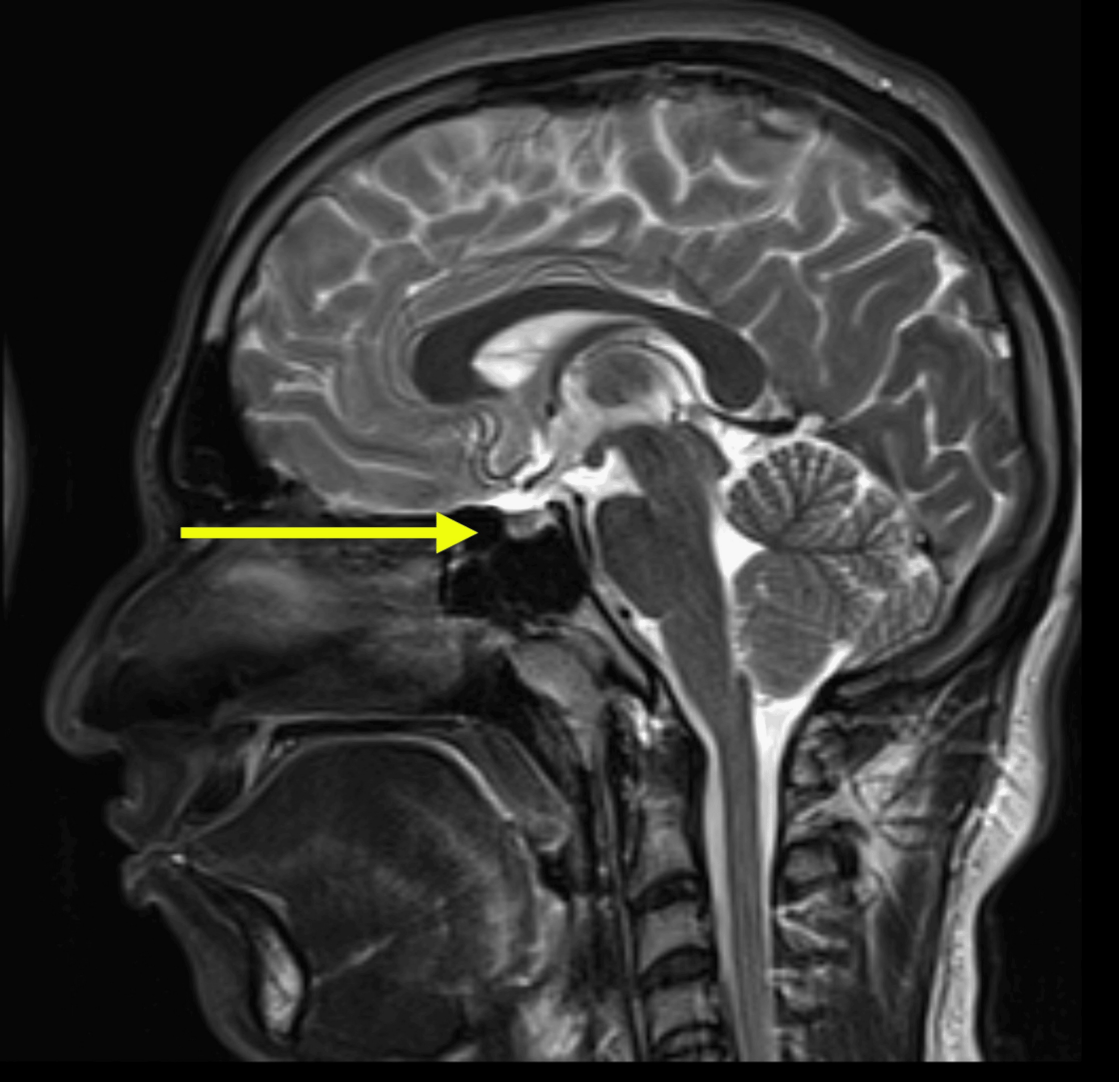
Sagittal T2‑weighted MRI of the brain showing the pituitary gland (yellow arrow) within the sella turcica. The pituitary stalk and optic chiasma are well visualised, with no abnormal signal changes or mass effect.
